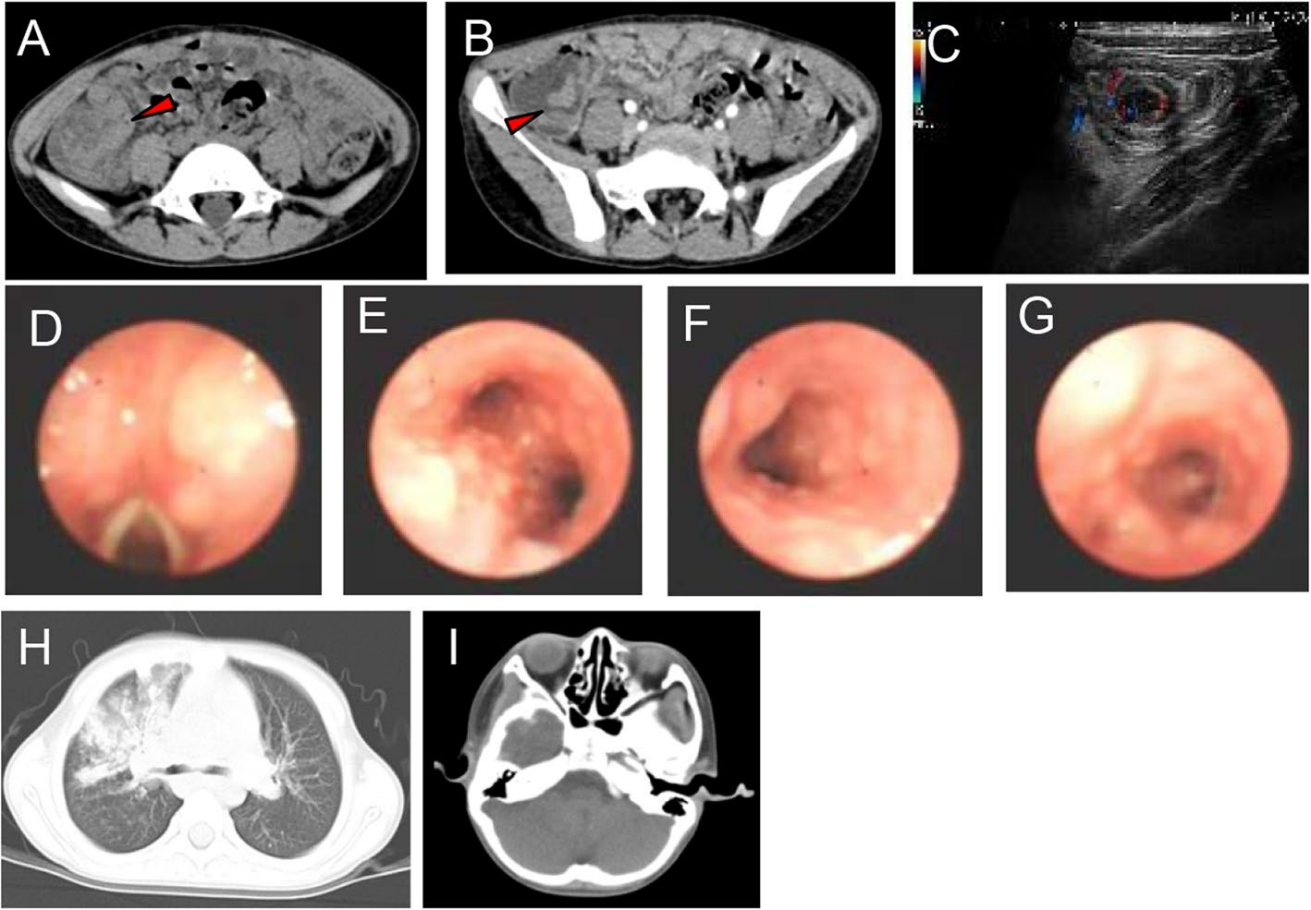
Clinical phenotype of patients with APDS1.  Ileocecal intussusception and polypoid soft tissue nodule in ileocecal intestinal cavity revealed by MRI (A and B) and target ring sign detected by ultrasound (C) in patient 2. Mucosal nodule lymphoid hyperplasia from pharynx to the entire airway revealed by bronchoscopy in patient 3 (D-G); Pulmonary parenchymal lesions and patchy infiltration, and maxillary sinusitis in patient 3 by CT scan (H, I)
